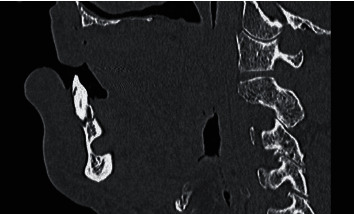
Computed tomography image, sagittal cross section, hard tissue mode. The lingual cortical bone recessed from the lingual to the buccal side is contiguous with the buccal cortical bone at the deepest point of the cavity. There is no connection of the apex of the adjacent #27 with the cavity in the lingual side of the mandible, and a high-density line suggests the presence of bony tissue between the apex of #27 and the cavity in the lingual side of the mandible.
